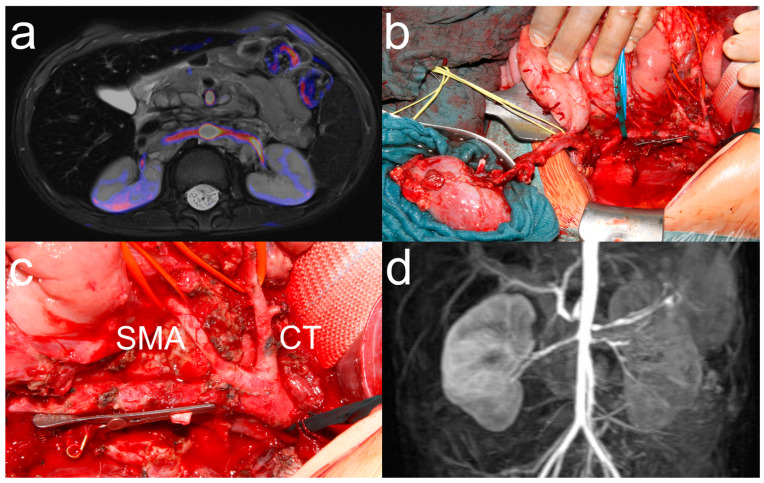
Renal autotransplantation for resection of high-risk neuroblastoma. A 5-year-old girl with high-risk neuroblastoma involving both renal arteries on preoperative image fusion of transversal T2-weighted sequence and MR angiography (a). Intraoperatively, the left renal vein had to be resected due to complete tumor infiltration. The kidney was mobilized ante situ (b) with the ureter left in place (yellow loop, blue loop: inferior vena cava). Aorta (c) with Yasargil clamp on the stump of the renal artery. The superior mesenteric artery (SMA) and coeliac trunk (CT) are slung with red vessel loops. Early postoperative angio-MRI showing slight irregularity of the left renal artery on MIP and consequently weaker contrast enhancement of the left kidney after renal autotransplantation (d).
